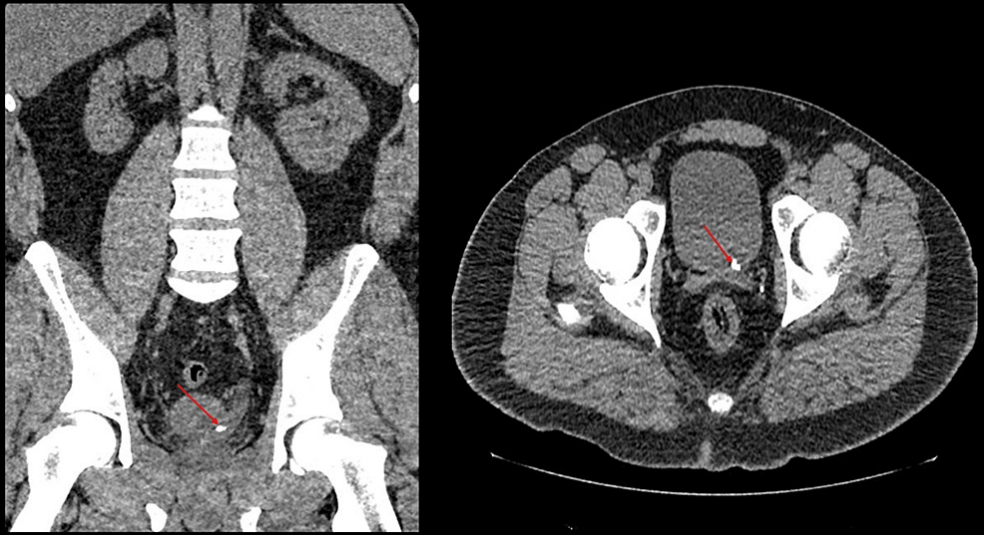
The first CTKUB shows the left VUJ stone measuring 6 mm. Left: coronal section; Right: axial view. The stone is labelled with red arrows. CTKUB: computed tomography of kidney, ureters and, bladder; VUJ: vesico-ureteric junction
